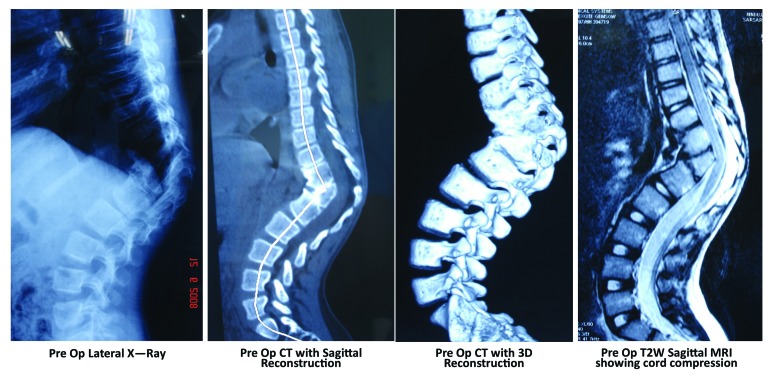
13 year old female patient with congential kyphosis with progressive paraparesis. Note the presence of extra pedicle with anterior aplasia and toppling in CT Scan. MRI shows cord compression.
